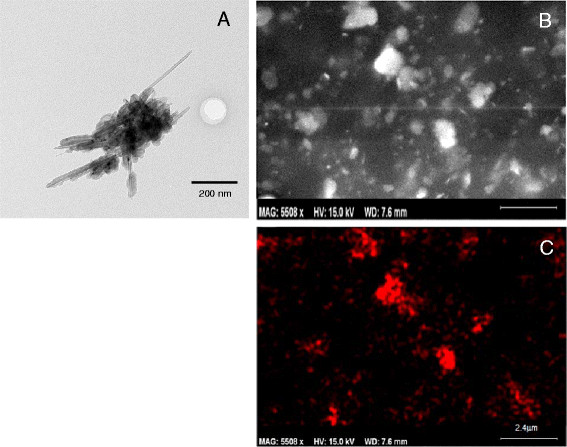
AO nanowhiskers collected in the exposure chamber. A TEM image (A), SEM image (B) and SEM EDS map (C) of the same region as shown in Figure 5B. Scale bars in 5B and 5 C represent 2.4 μm. Red color represents signal collected at characteristic X-ray energy for Al metal.
